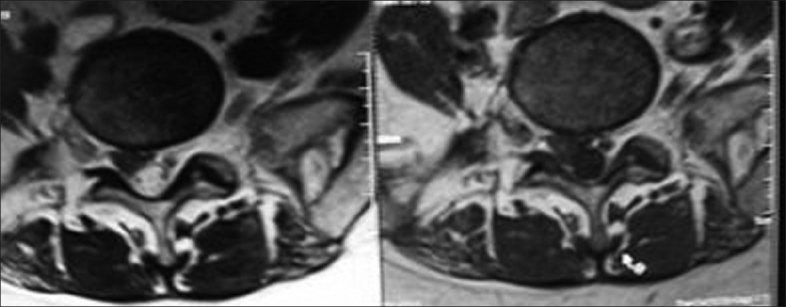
Axial sections of MRI lumbar spine showing right sided disc herniation compressing the traversing nerve root
